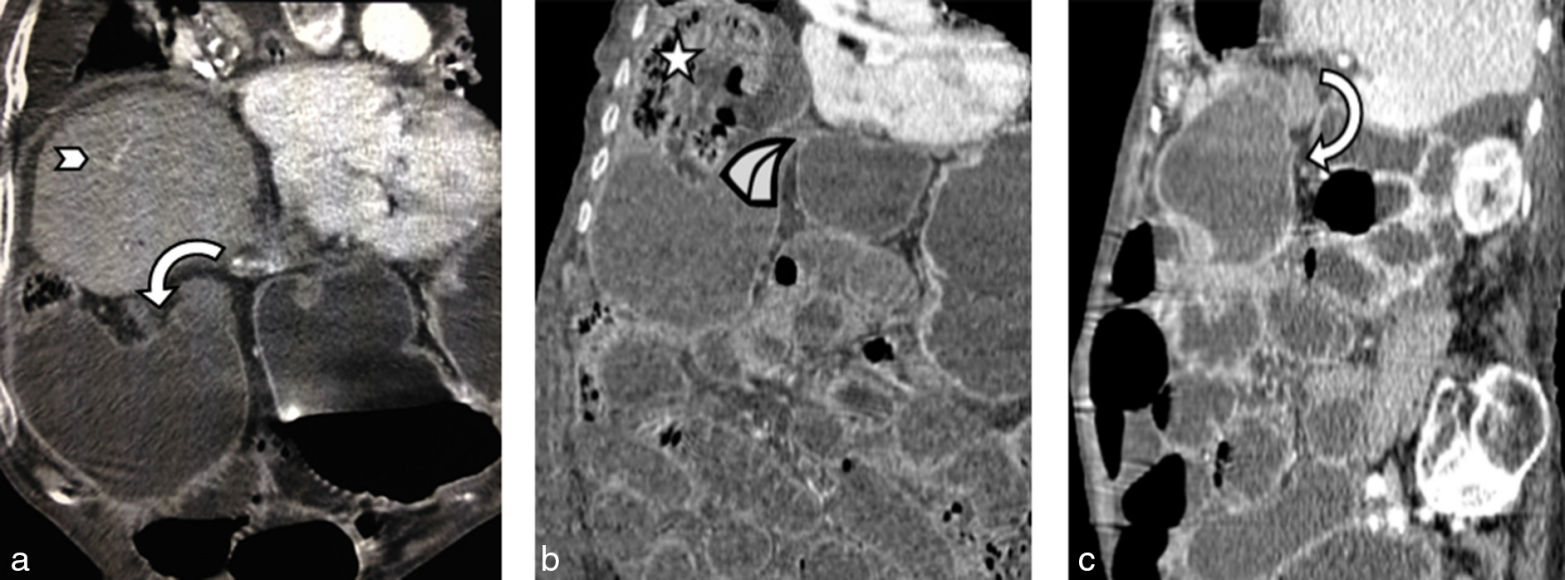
CT multiplanar reconstructions demonstrating the crucial beak and swirl features of gallbladder volvulus. (a) Coronal oblique reconstruction. There is gross distension of the gallbladder, which is in a horizontal lie. There is abrupt angulation at the Hartmann’s pouch (curved arrow). The arrowhead denotes the right lobe of the liver. (b) Coronal reconstruction. Angulation at the Hartmann’s pouch gives rise to a “beak” sign (highlighted with a sketch of a beak). Note the incidental Chilaiditi’s sign (star). (c) Sagittal image. Curved arrow demonstrates a mini “swirl” sign at the point of angulation, which represents a gallbladder volvulus (curved arrow).
